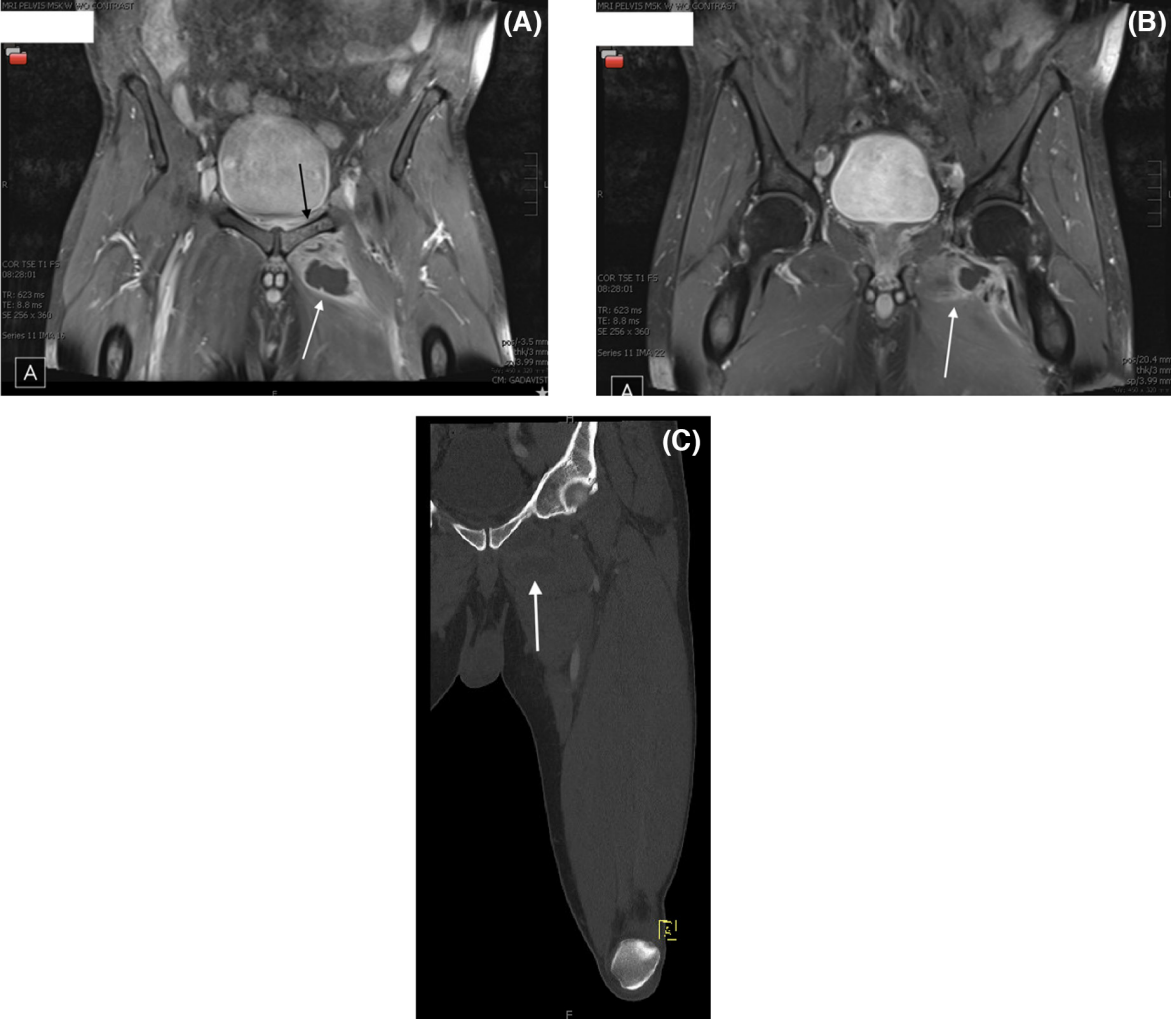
(A–C) Magnetic resonance imaging (MRI) with and without contrast of the pelvis showing recurrent abscesses (white arrow), with new findings concerning for pelvic osteomyelitis (black arrow).
